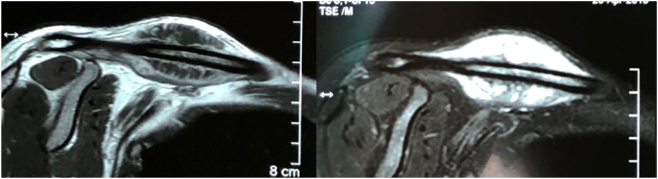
MRI showed hypo-intense signal lesion on T1W, hyper-intense on T2-W sequences and heterogenous enhanced with contrast. Noted that intramedullary hyper-intense on T2.
